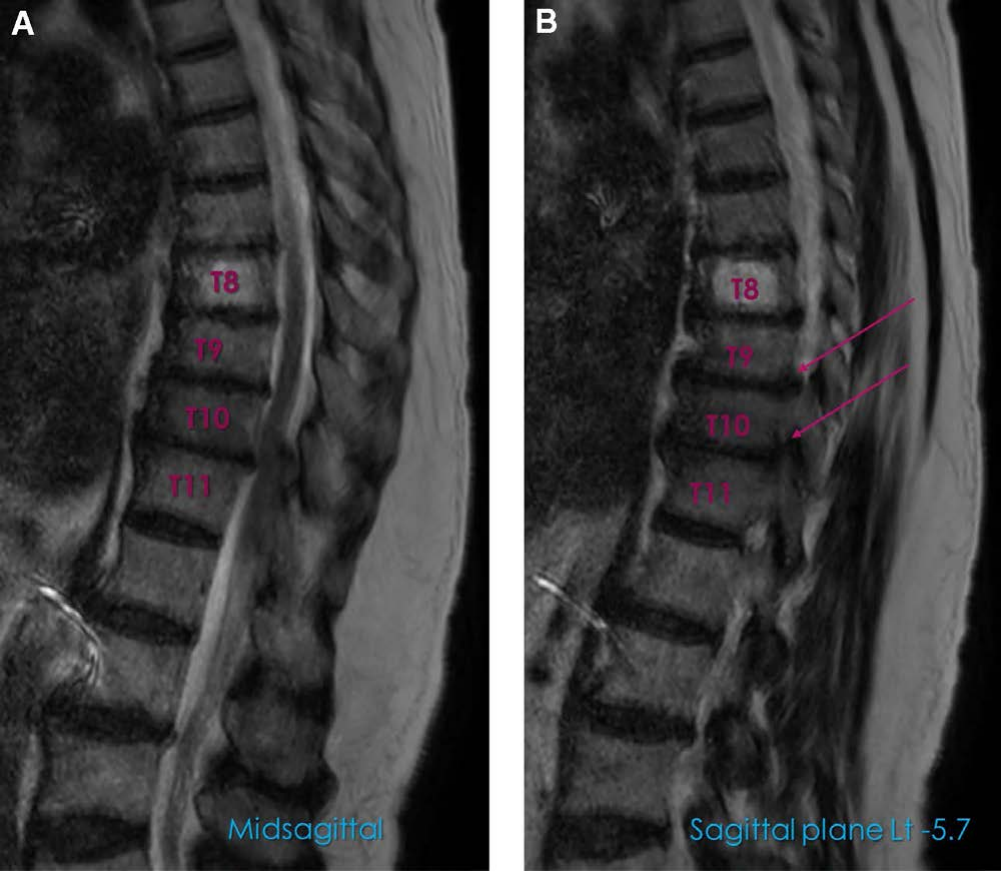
Sagittal whole-spine magnetic resonance T2-weighted imaging showing multilevel HTD at T8/9/10/11. (A) Midsagittal plane. (B) Sagittal plane suggesting foraminal herniated disc at left T9/10/11. HTD = herniation of the thoracic intervertebral disc.
